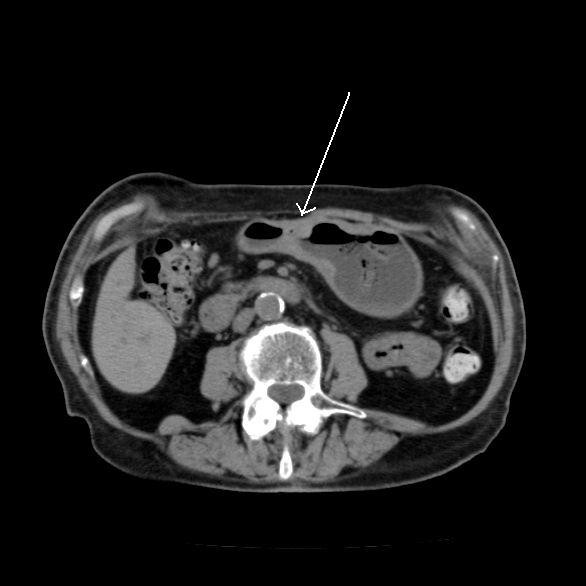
Preoperative CT scan. It shows the gastric adenocarcinoma of the antrum, involving both anterior and posterior gastric walls.
